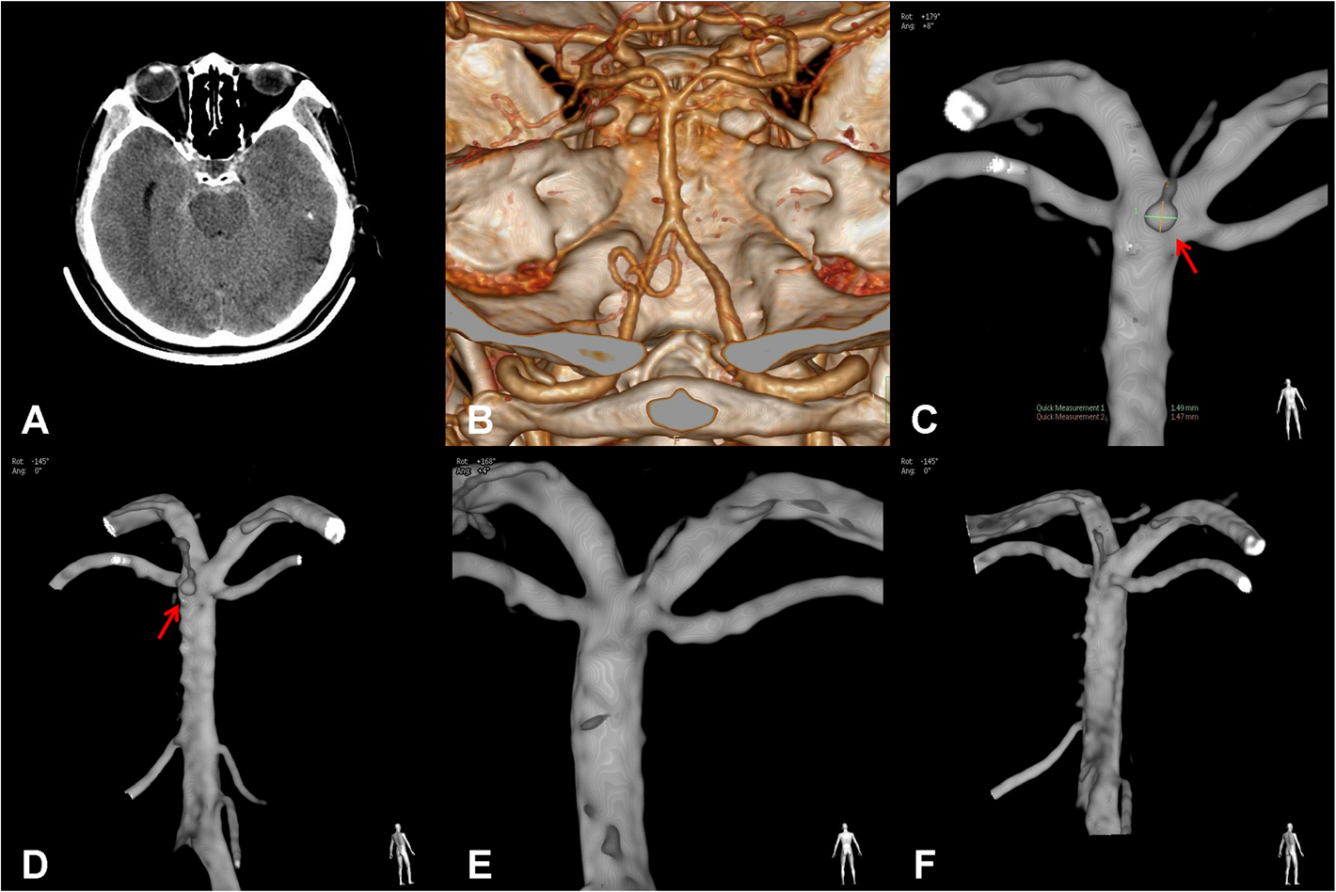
Imaging data of the case. A SAH surrounding potine was confirmed by head CT after onset. B Emergency CTA did not demonstrate definite source of hemorrhage. C, D Three-dimensional rotation angiography (3DRA) revealed a small aneurysm localized at posterior perforator of rostral basilar trunk. E, F The lesion disappeared from DSA during 2 months of follow-up with preservation of parent perforator
